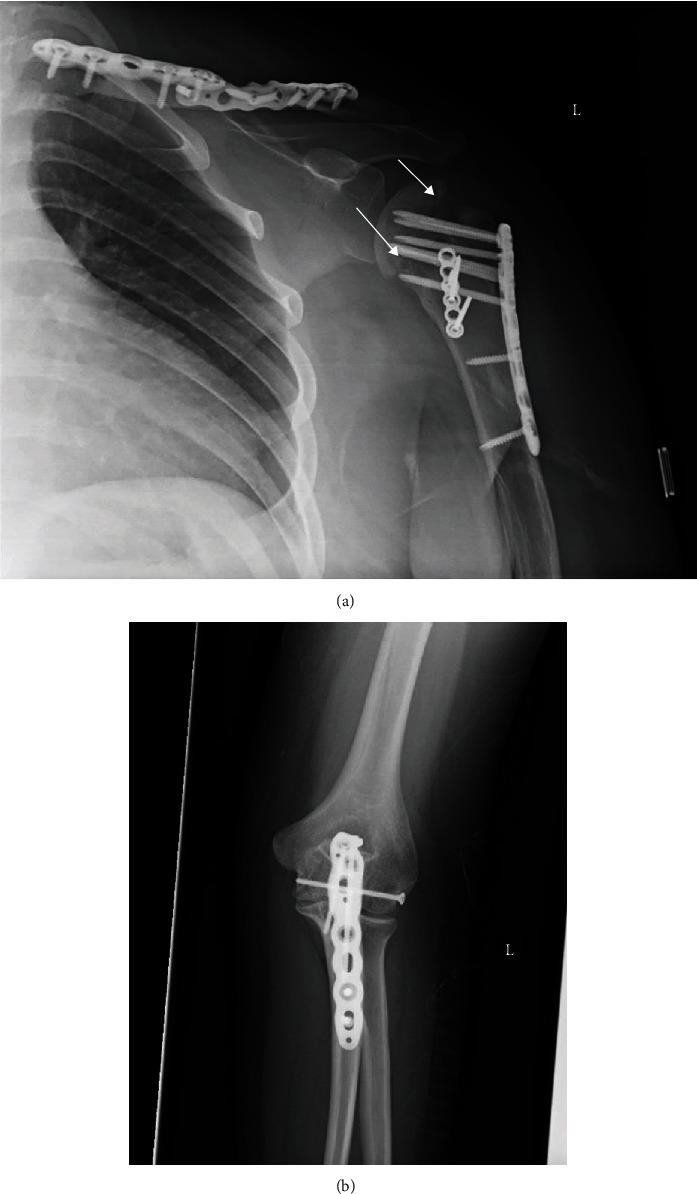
(a) Anteroposterior X-ray of the left shoulder and (b) anteroposterior X-ray of the left olecranon process reveal proper alignment of fractures with subchondral lucency (arrows) seen in the humeral head suggestive of mild avascular necrosis after nine months postsurgical intervention.
